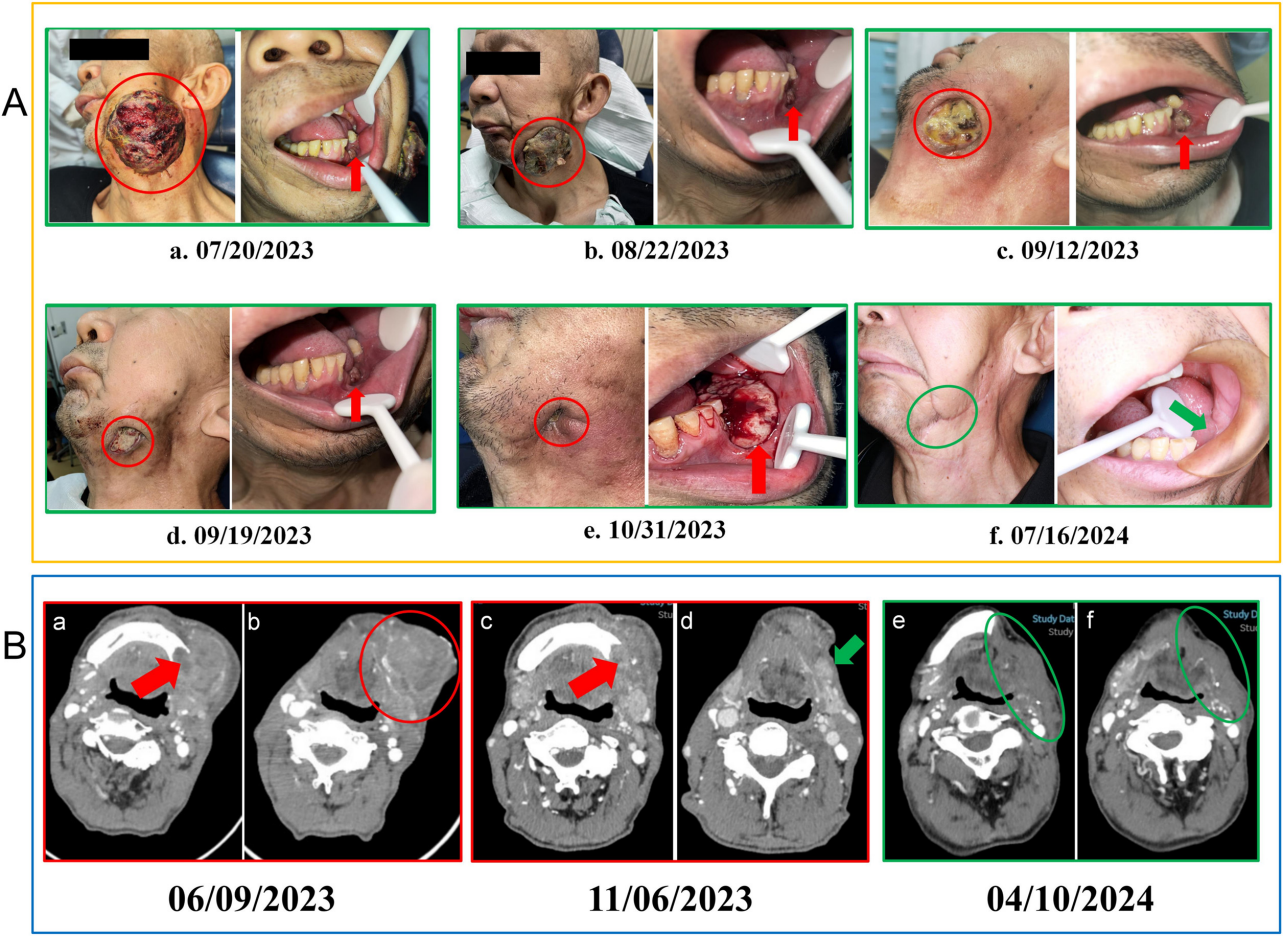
(A) Changes in the lesions during treatment. (a) Prior to treatment, the intraoral lesion displayed tooth loss in the left lower molar region with destruction of the alveolar ridge, while the left submandibular metastatic lesion showed surface ulceration and bleeding. (b) After two cycles of treatment, the intraoral lesion showed minor reduction, and the left submandibular metastatic lesion decreased significantly. (c) Nine days after three cycles, the intraoral lesion remained relatively unchanged, while the submandibular lesion demonstrated showed further reduction. (d) Sixteen days after three cycles, the intraoral lesion remained stable, and the submandibular lesion maintained a PR status. (e) Fourteen days after five cycles of treatment, the tumour in the left lower gingiva showed rapid growth, while the submandibular lesion had nearly disappeared. (f) Five months post-surgery, no recurrence of the lesion was observed. Red circles mark the extraoral lesion, red arrows point to the intraoral lesion, while green arrows and circles indicate the intraoral and extraoral wound status 5 months post-surgery. PR, partial response; CT, computed tomography; (B) Patient imaging examinations during the course of the disease. (a, b) At the time of initial diagnosis, a CT scan of the neck revealed osteolytic destruction of the left mandibular body and ramus, along with left-sided lymphadenopathy. (c, d) Following five cycles of combined immune-targeted therapy, no significant change was noted in the bony destruction, but the surrounding soft tissue mass had reduced. (e, f) A follow-up CT scan on April 10, 2023, five months post-surgery, showed no signs of recurrence. Red arrows indicate the mandibular destruction area, red circles mark the submandibular metastatic lesion, green arrows show the submandibular lesion after 5 cycles of combined targeted therapy, and green circles represent the original lesion site 5 months post-surgery.
